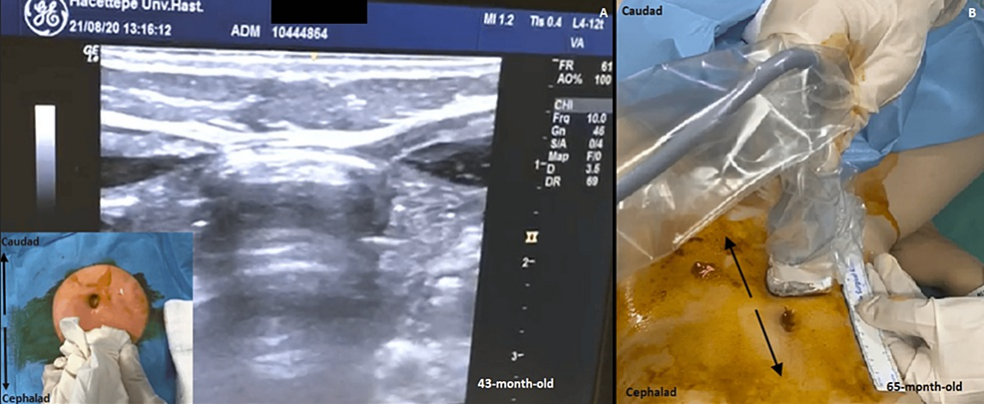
The measurement of spread distance by sliding the ultrasound probe toward the cephalad and caudad directions from the injection point. (A) Epigastric hernia repair of a patient at 43 months of age with the ultrasound probe at the injection point. (B) Laparoscopic surgery of a patient at 65 months of age showing the measurement technique.
